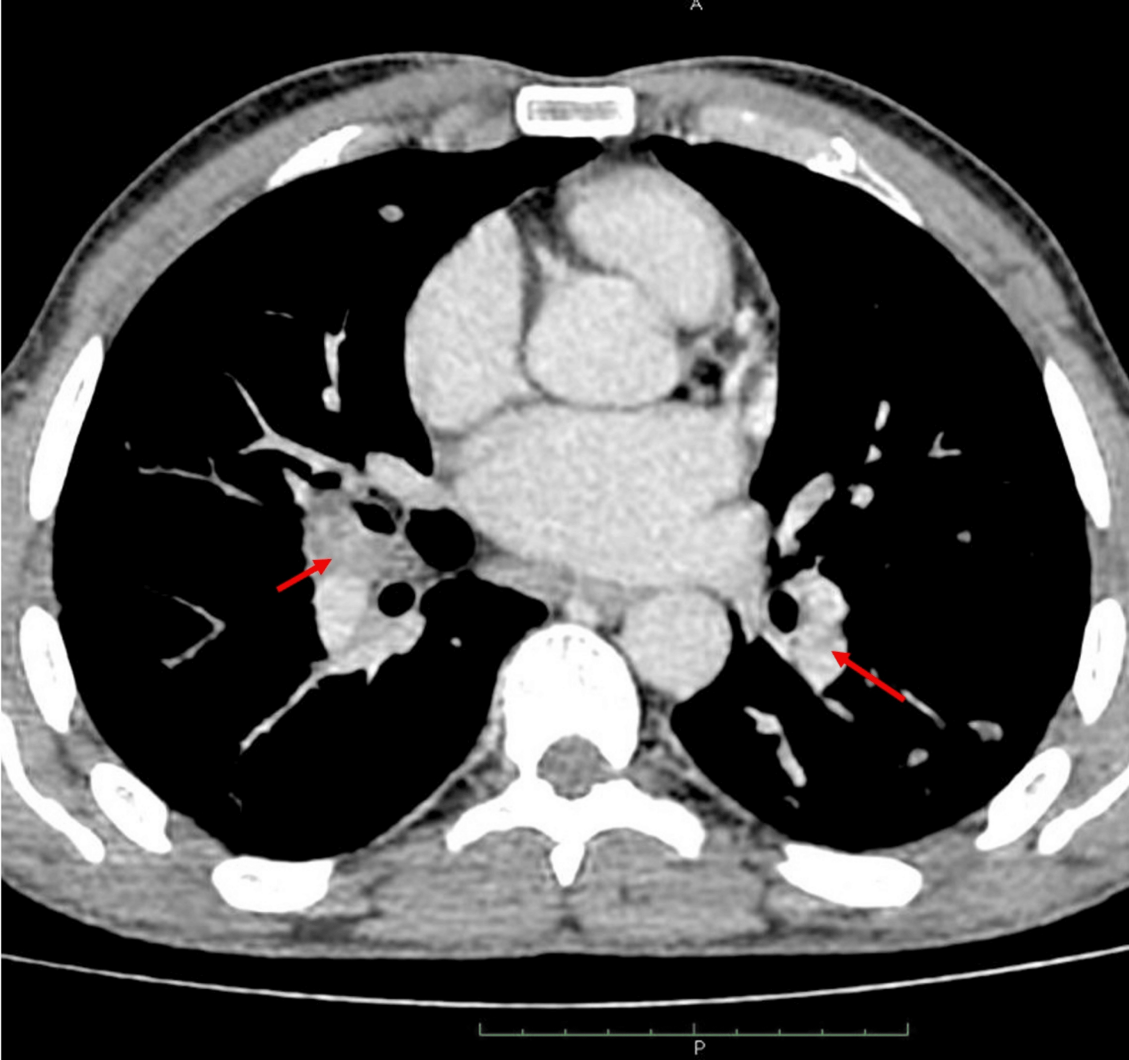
Thoracic CT scan showed multiple bilateral, non-compressive and non-necrotic mediastinal lymphadenopathy (red arrows).
